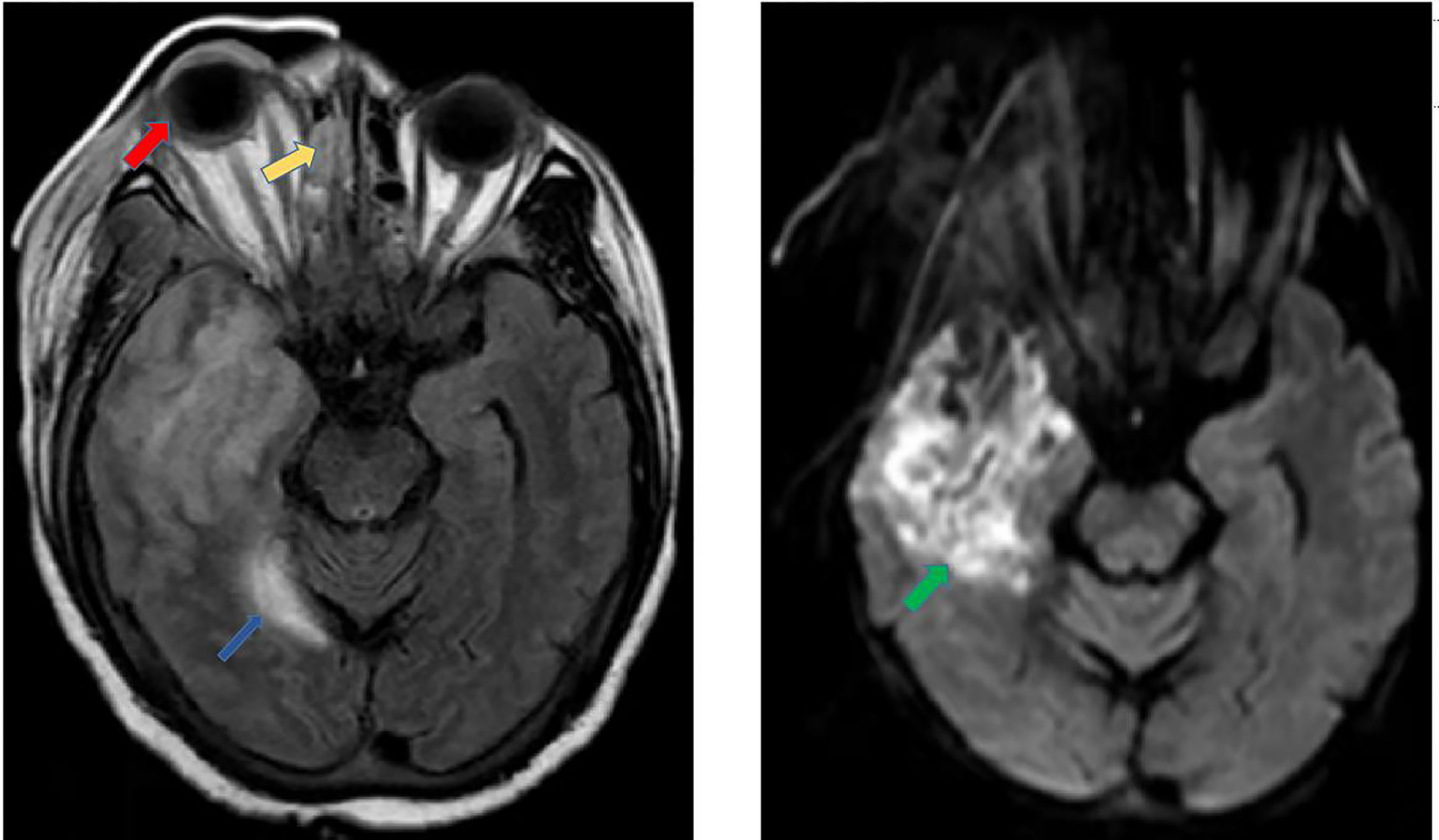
Enhanced magnetic resonance imaging and diffusion weighted imaging. The MRI results show that multiple recent infarcts in the right frontal and temporal regions (green arrow), protrusion of the right eyeball (red arrow), ethmoid sinusitis (yellow arrow), and a few paratentorial subdural/epidural hematoma (blue arrow).
